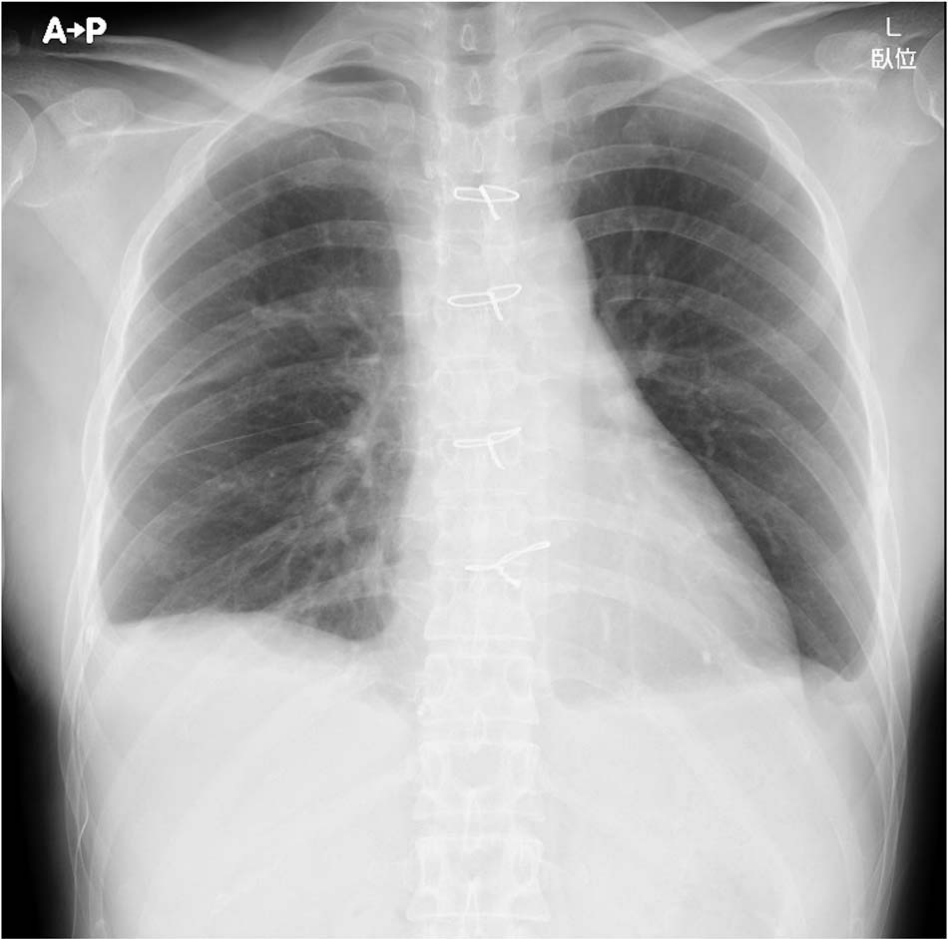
Chest X-ray on post-operative day 7 revealing no massive pleural effusion.
